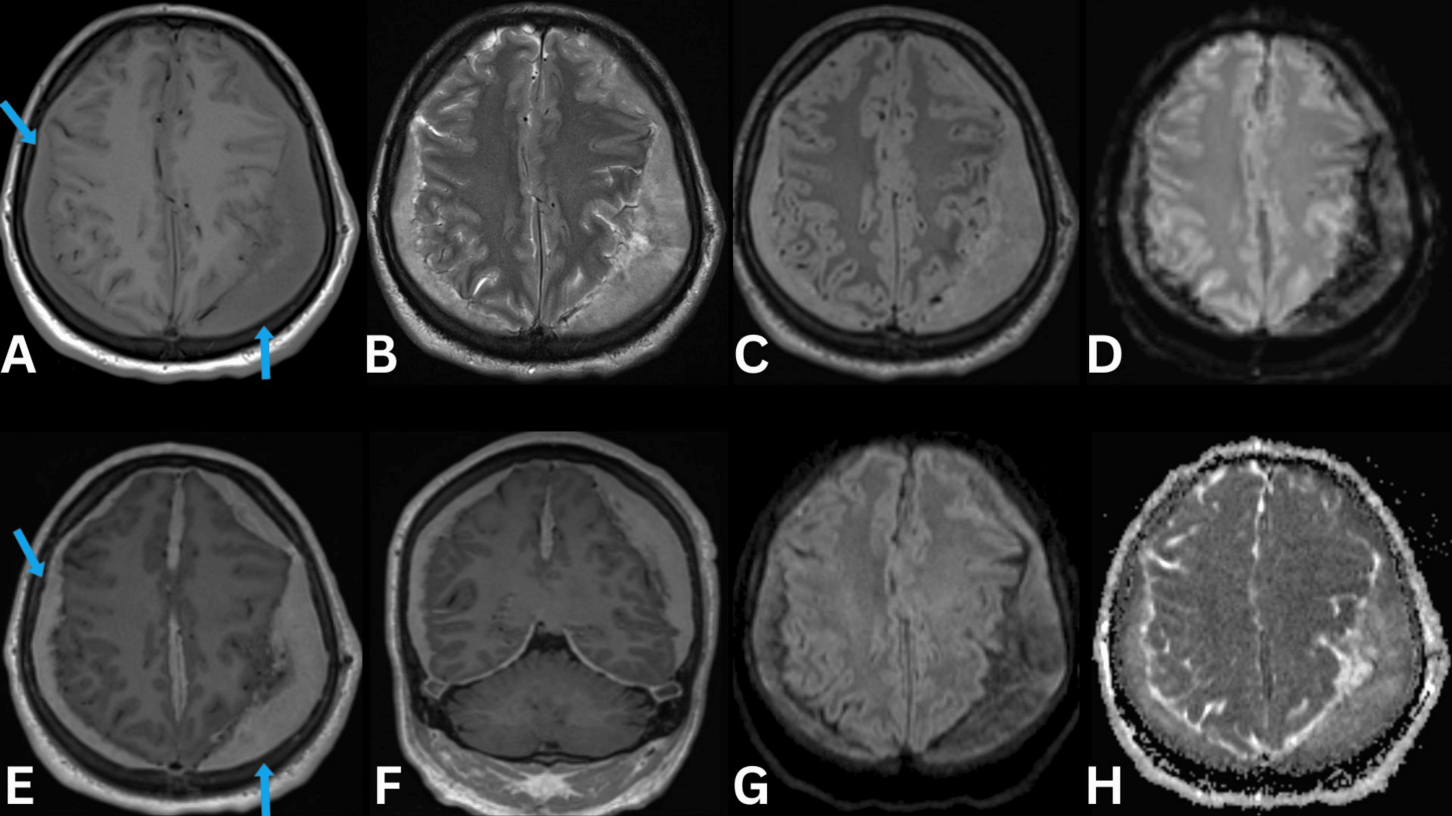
MRI brain with and without contrast T1-weighted axial view (A), T2-weighted axial view (B), FLAIR axial view (C), DWI axial view (D), T1-weighted post-contrast axial view (E), T1-weighted post-contrast coronal view (F), SWI axial view (G), and ADC axial view (H) reveal extra-axial lesions bilaterally along the cerebral convexities showing uniform mild post-contrast enhancement (blue arrows). These show iso to slightly hypointense T1 and heterogeneous T2 signal intensity, relatively high signal intensity on the FLAIR image, and susceptibility artifact on the SWI image, suggesting hemosiderin disposition. There is no diffuse restriction within the lesions. DWI: diffusion-weighted imaging; SWI: susceptibility-weighted imaging; ADC: apparent diffusion coefficient; FLAIR: fluid-attenuated inversion recovery
